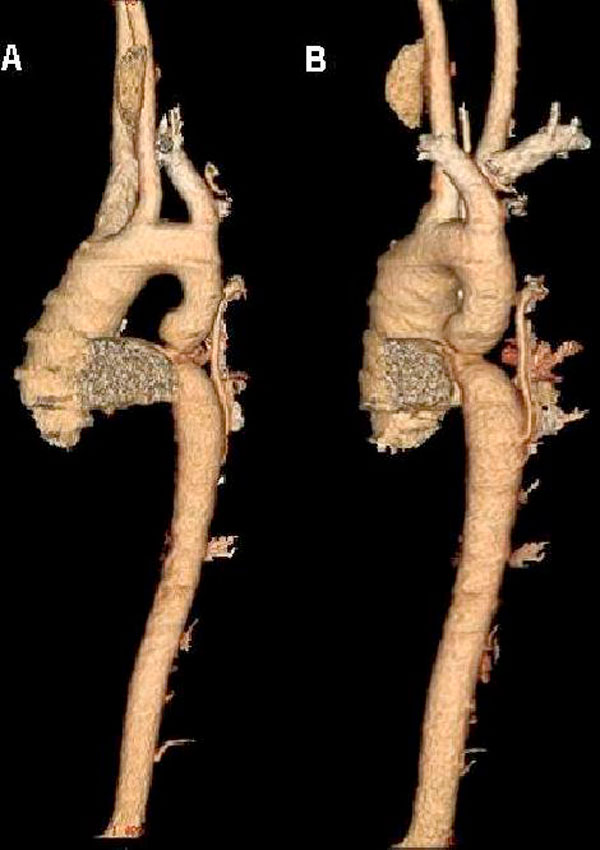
Thorax CT angiography in anterior posterior (A) and left anterior oblique projections (B), a strict aortic coarctation proximal part of descending aort, ascending aort dilatation, a small size patent ductus arteriosus, and widening and tortuous intercostals artery was detected.
